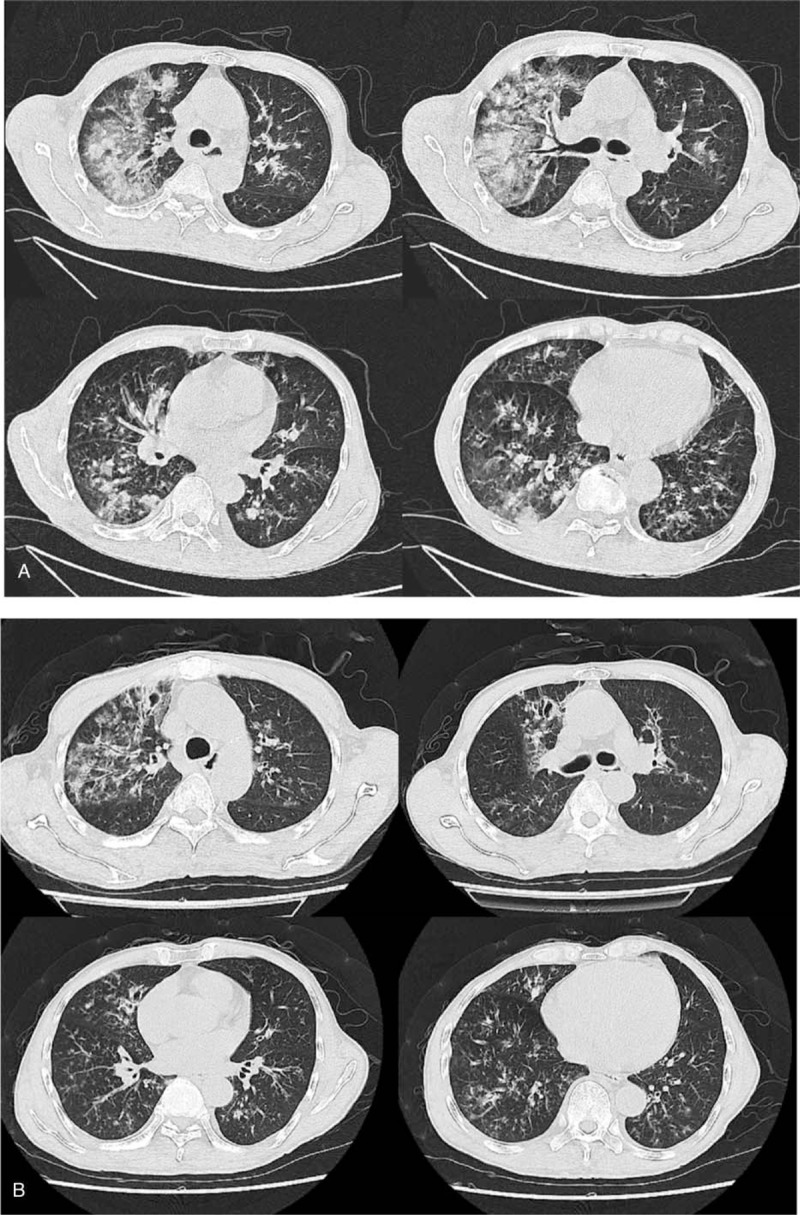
A. HRCT of the chest showing inflammation in both lungs with central bronchiectasis; B. HRCT of the chest showing improved inflammation of both lungs after antifungal treatment. HRCT = high-resolution computed tomography.
